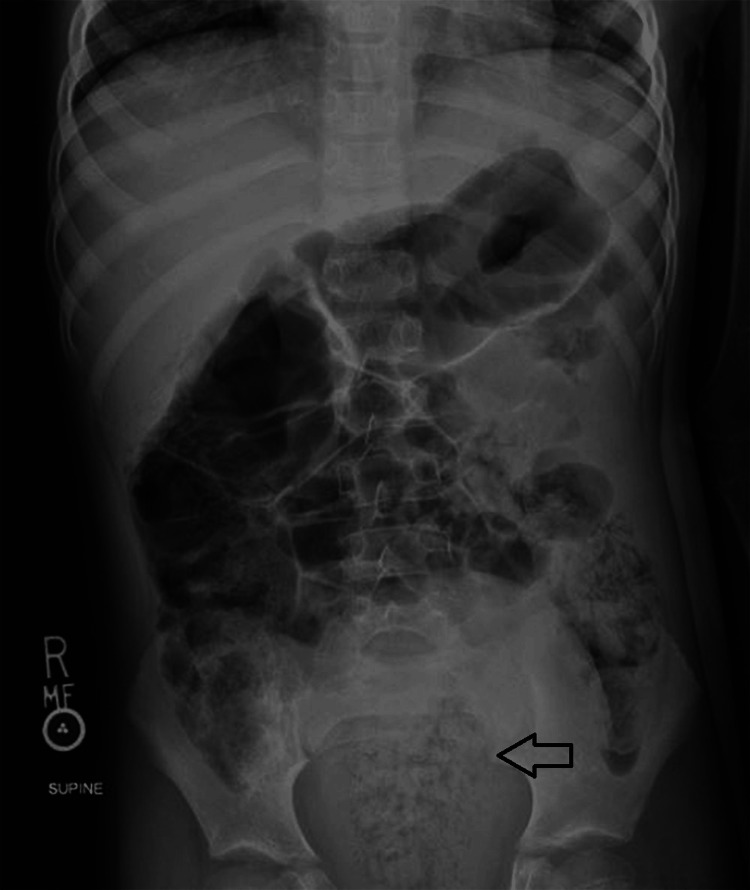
Abdominal X-ray on admission demonstrating moderate stool burden in the colon with a large stool burden in the rectal vault of 1.89 in (4.8 cm) that is identified by the black arrow
